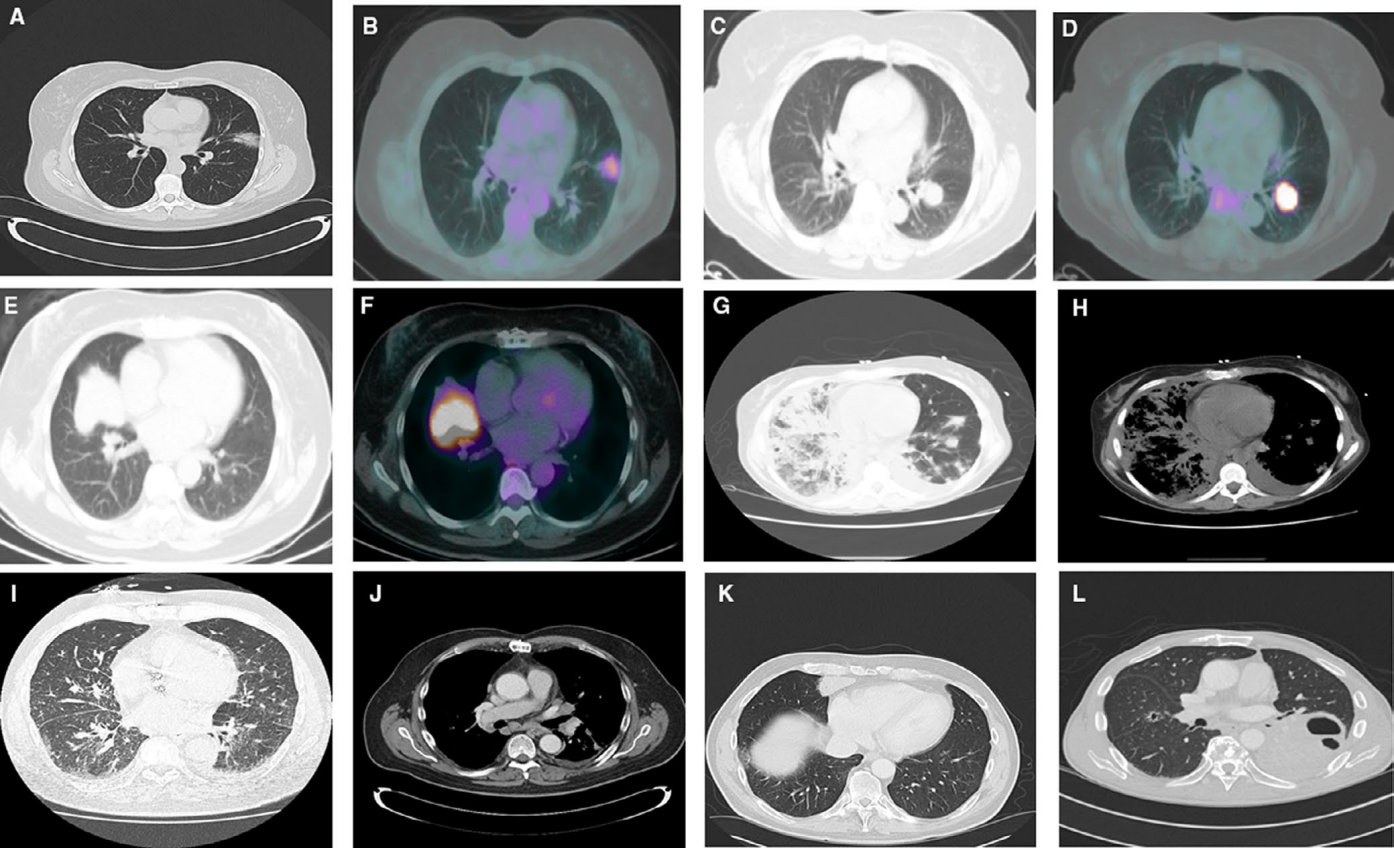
Radiological manifestations of pulmonary marginal zone lymphoma. A and B, focal ground glass opacity with air bronchograms and mild FDG‐uptake in the left upper lobe; (C and D), nodular lesion with intense FDG‐uptake in the left lower lobe; (E and F), pulmonary mass with intense FDG‐uptake in the right upper lobe; (G and H), multifocal bilateral lung parenchymal opacities with small left pleural effusion; (I and J), nodular interstitial thickening indicating lymphangitic spread of lymphoma, also noted to have mediastinal and hilar adenopathy on CT with contrast; (K), focal area of consolidation with homogeneous enhancement in the right middle lobe on CT with contrast; (L), cavitary consolidation in the left lower lobe with an additional cavitary nodule in the right middle lobe
